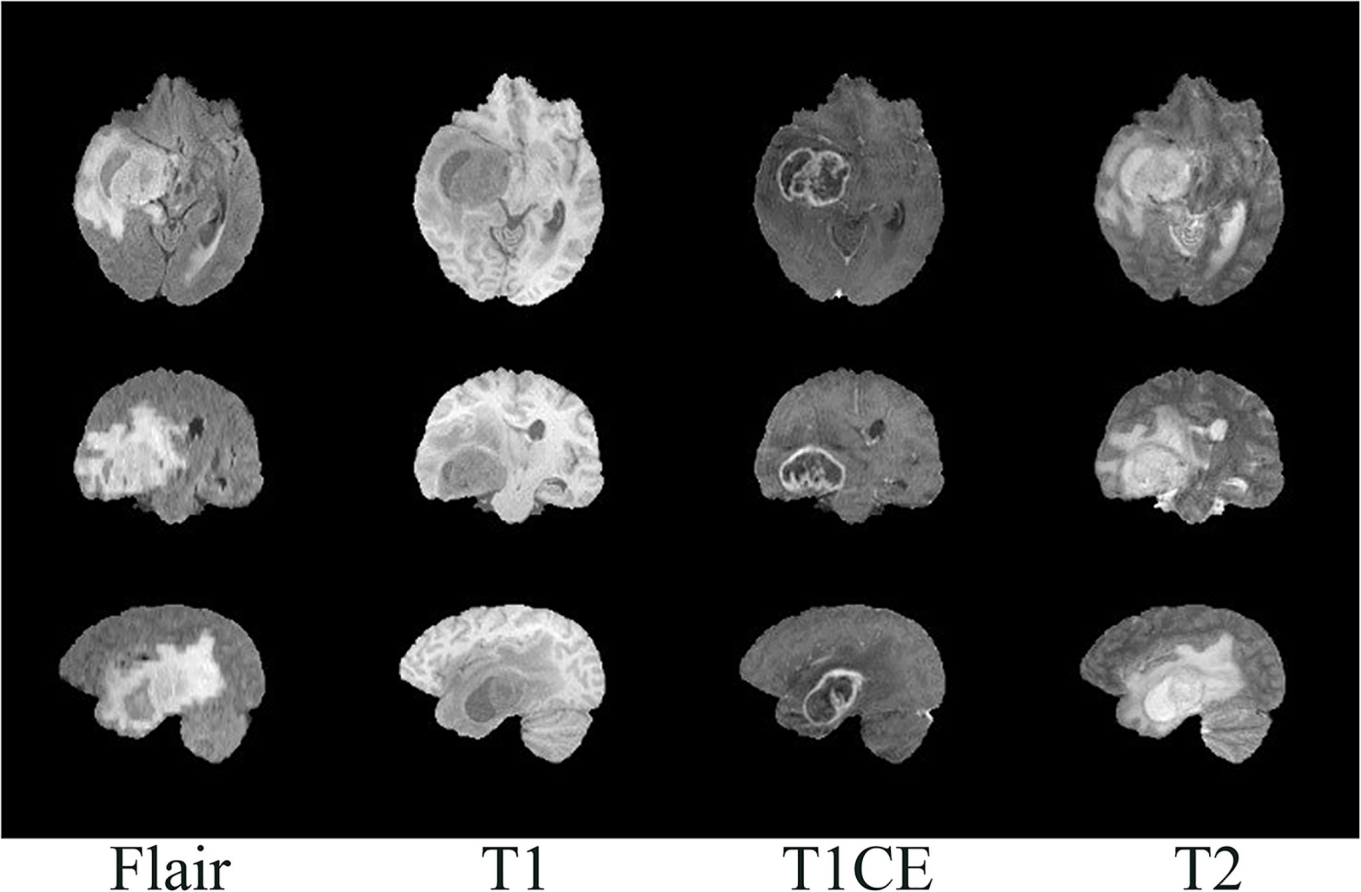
MRI images in 4 sequences and 3 sections. The first column represents the flair; the second column represents the T1; the third column represents the T1CE; the fourth column represents the T2, the first row represents the transverse plane; the second row represents the coronal plane; the third row represents the sagittal plane.
